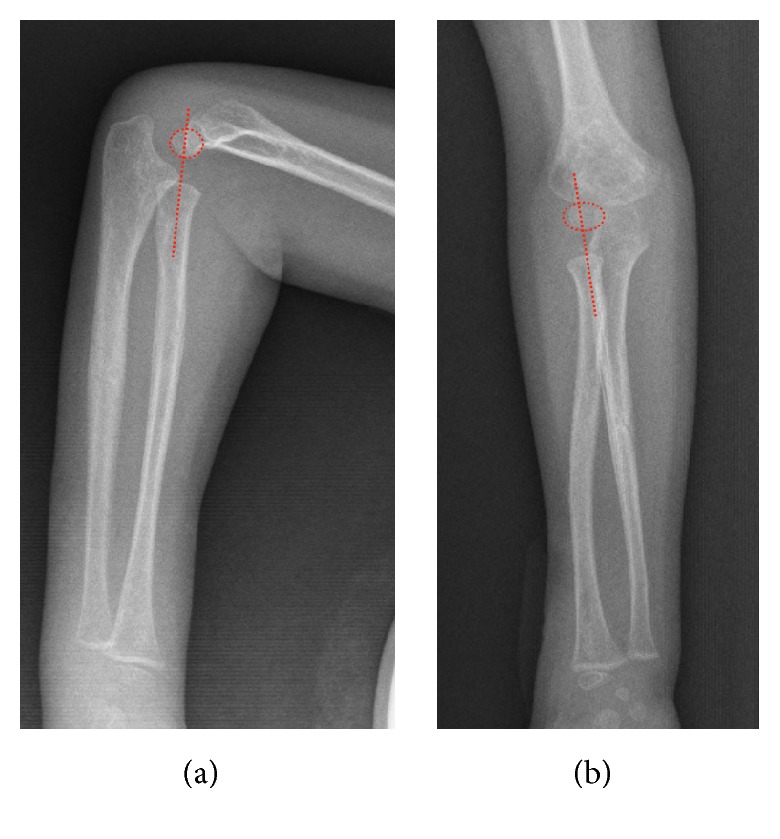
Radiographs after K-wire removal show successful union and reduction at the 6th week.
